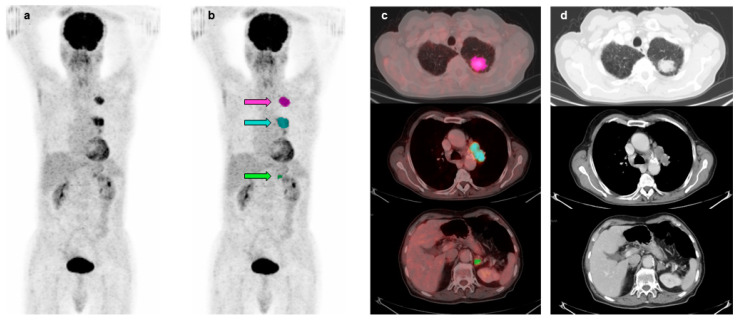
Representative images of ^18^F-FDG PET/CT scan in an 80-year-old patient with stage IVA NSCLC. Maximal intensity projection views without (a) and with an overlay of MTVs on primary tumor (pink arrow), lymph node (light blue arrow) and adrenal metastasis (green arrow) (b). Transaxial fusion images with overlay of MTVs on primary tumor (pink), lymph node (light blue) and adrenal metastasis (green) (c). Corresponding transaxial CT images (d). MTV_TOT_ = 42.67 mL.
